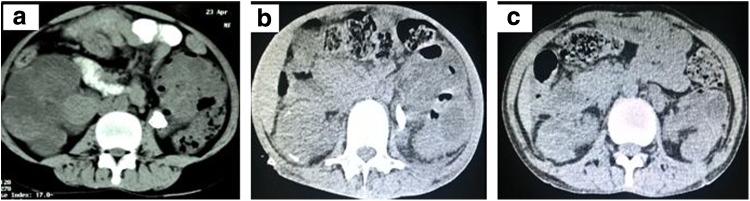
(a) Noncontrast computed tomography (NCCT) scan of the abdomen at presentation. (b) NCCT scan of the abdomen after amphotericin B therapy. (c) NCCT scan of the abdomen after nephroscopic clearance.
